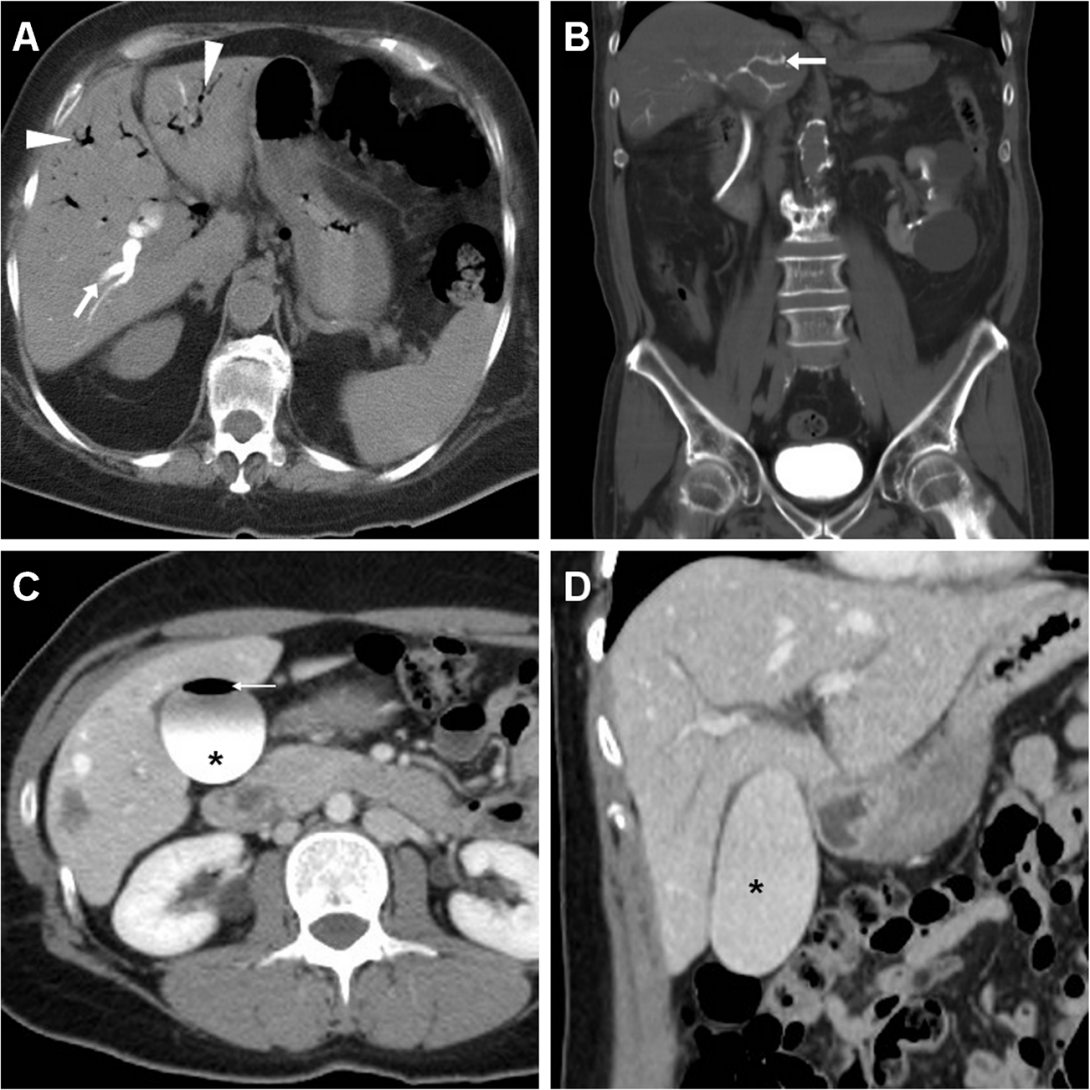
Normal findings observed hours after operative ERCP in a 75-year-old male investigated with unenhanced CT (c, d) to verify correct placement of a plastic biliary stent, including dependent contrast medium (*arrows*) and air (*arrowheads*) in the intrahepatic bile ducts. In a different patient, a 44-year-old female patient suffering from acute post-procedural pain, contrast-enhanced CT (a, b) 2 days after ERCP excluded signs of acute complications and showed stratified iodinated contrast medium (*), bile and air (*thin arrow*) in the gallbladder
